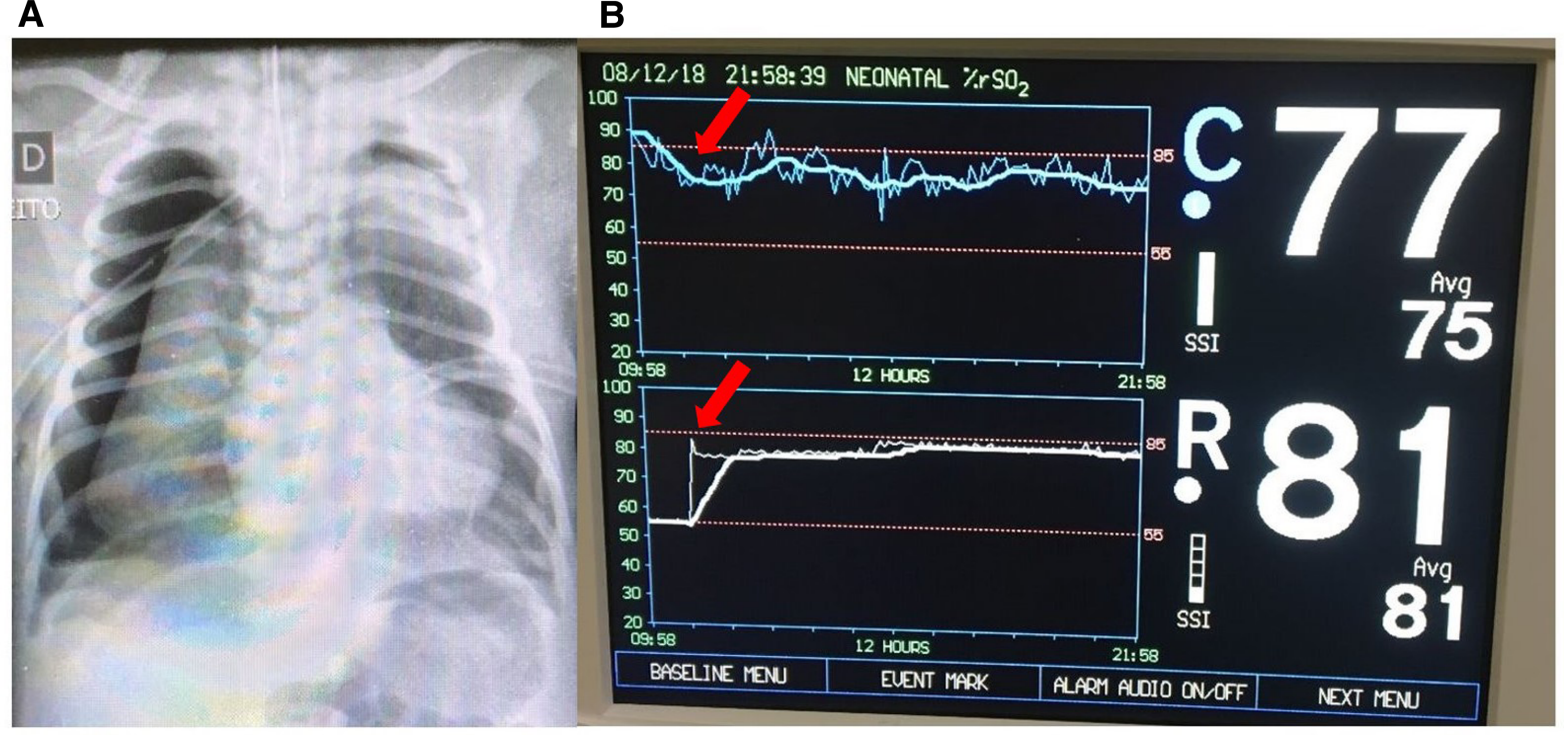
(A) Chest x-ray showing bilateral pneumothoraces. (B) Improvement of NIRS findings immediately after pneumothorax treatment with needle aspiration (arrows). The rScO_2_ (blue trend line) decreased to normal levels (∼75% to 77%) and rSrO_2_ (white trend line) increased to 81%.
